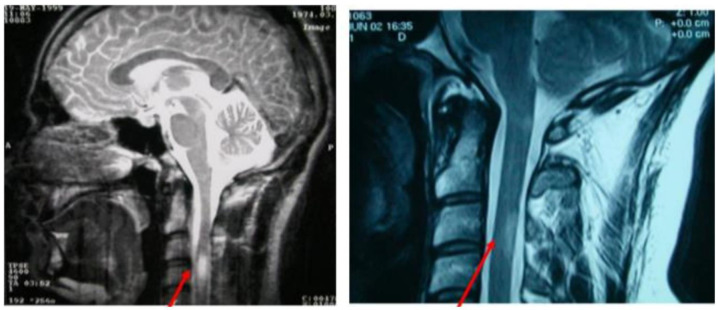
Spinal cord lesions on MRI.
